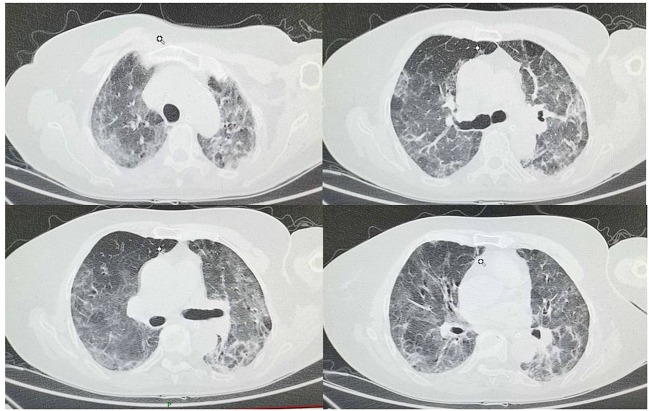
On March 4th chest CT shows less bilateral interstitial ground glass-like shadows and multiple consolidations compared to chest CT on February 20th.
